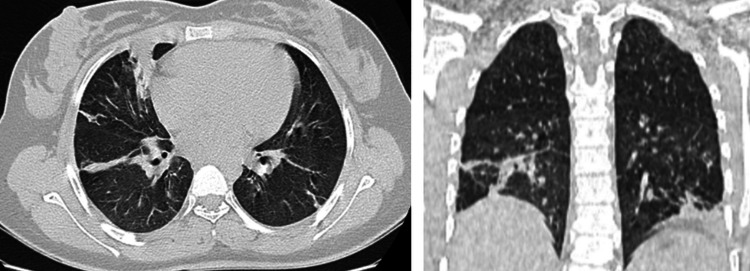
Chest CT one month later. Presence of reticulations and thickening of interlobular septa. Residual left basal consolidation and a perihilar consolidation on the right. Complete resolution of bronchial dilatation, suggesting regression of pseudocysts associated with *Pneumocystis jirovecii*.
